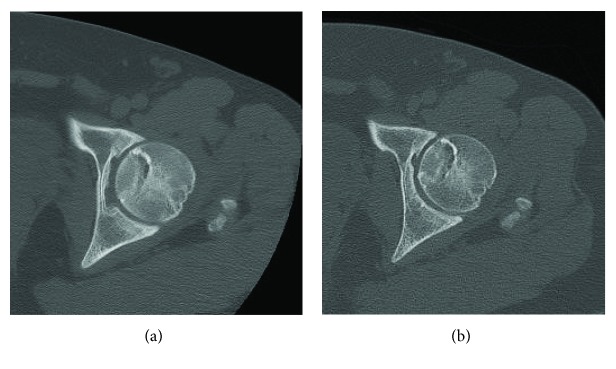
Axial CT-Scan of the left hip: (a) 2 years postoperatively and (b) 6 years postoperatively.
